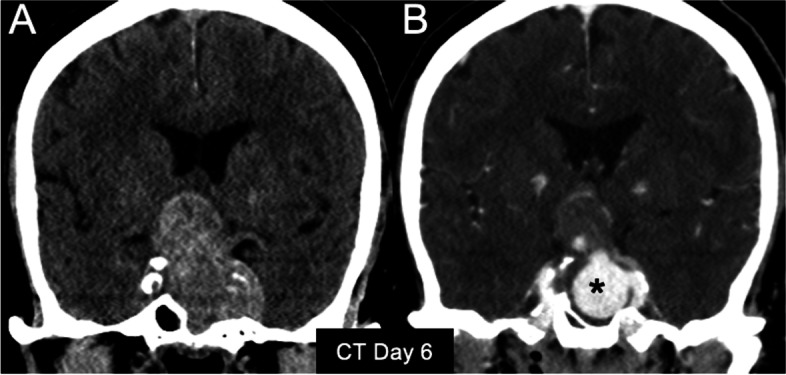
A Unenhanced and (B) enhanced computed tomography (CT) images obtained from the outside hospital soon after the patient experienced the second attack of severe headache. B A vividly enhancing pouch (asterisk) on contrast-enhanced CT imaging appeared to communicate with the left cavernous internal carotid artery. The findings were highly suggestive of intratumoural pseudoaneurysm formation, although the outside CT report indicated an enlarging apoplectic macroadenoma
